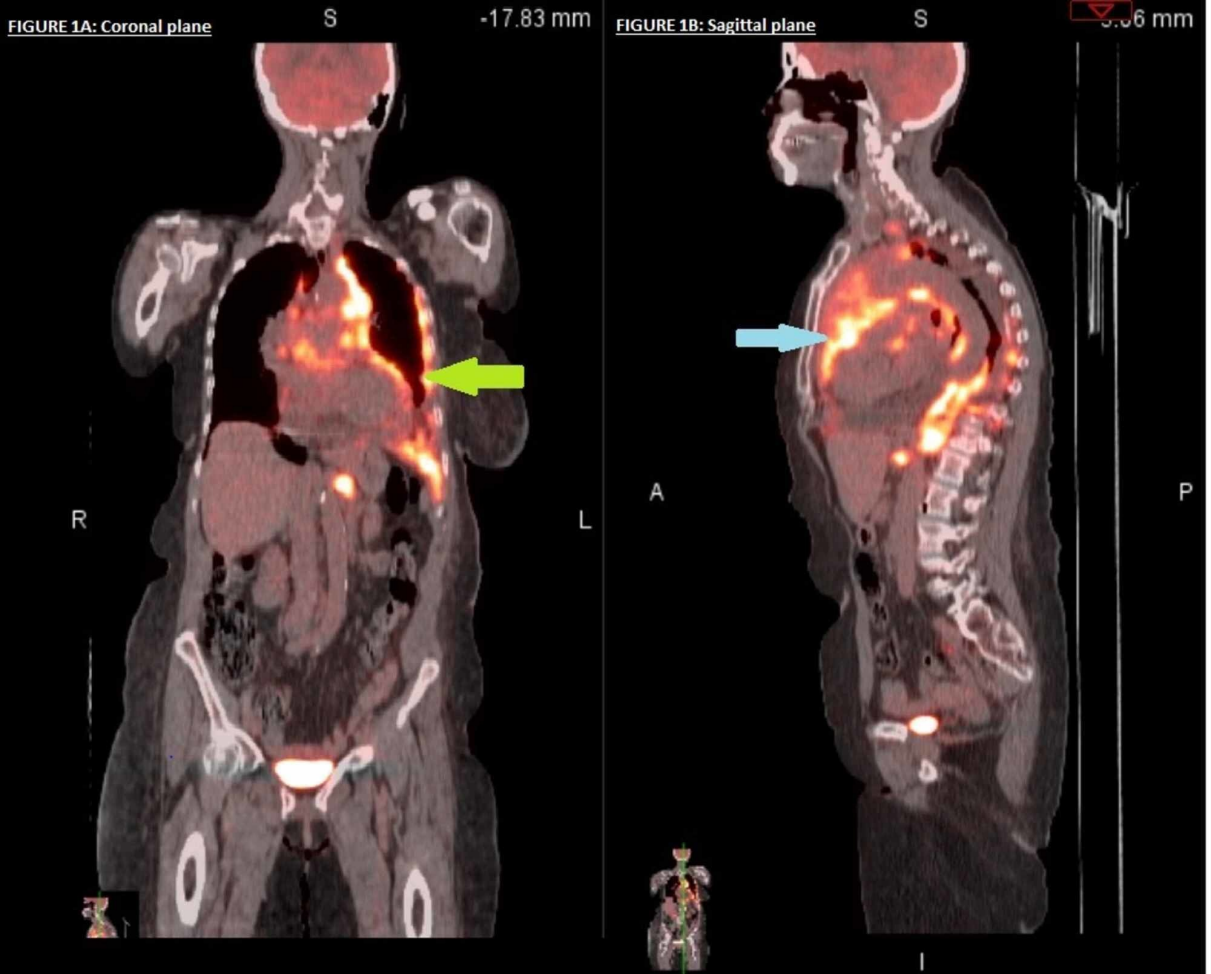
Positron emission tomography (PET)/CT showing hypermetabolic marked pleural thickening throughout the left hemithorax periphery (green arrow) and anterior mediastinal mass (blue arrow).
